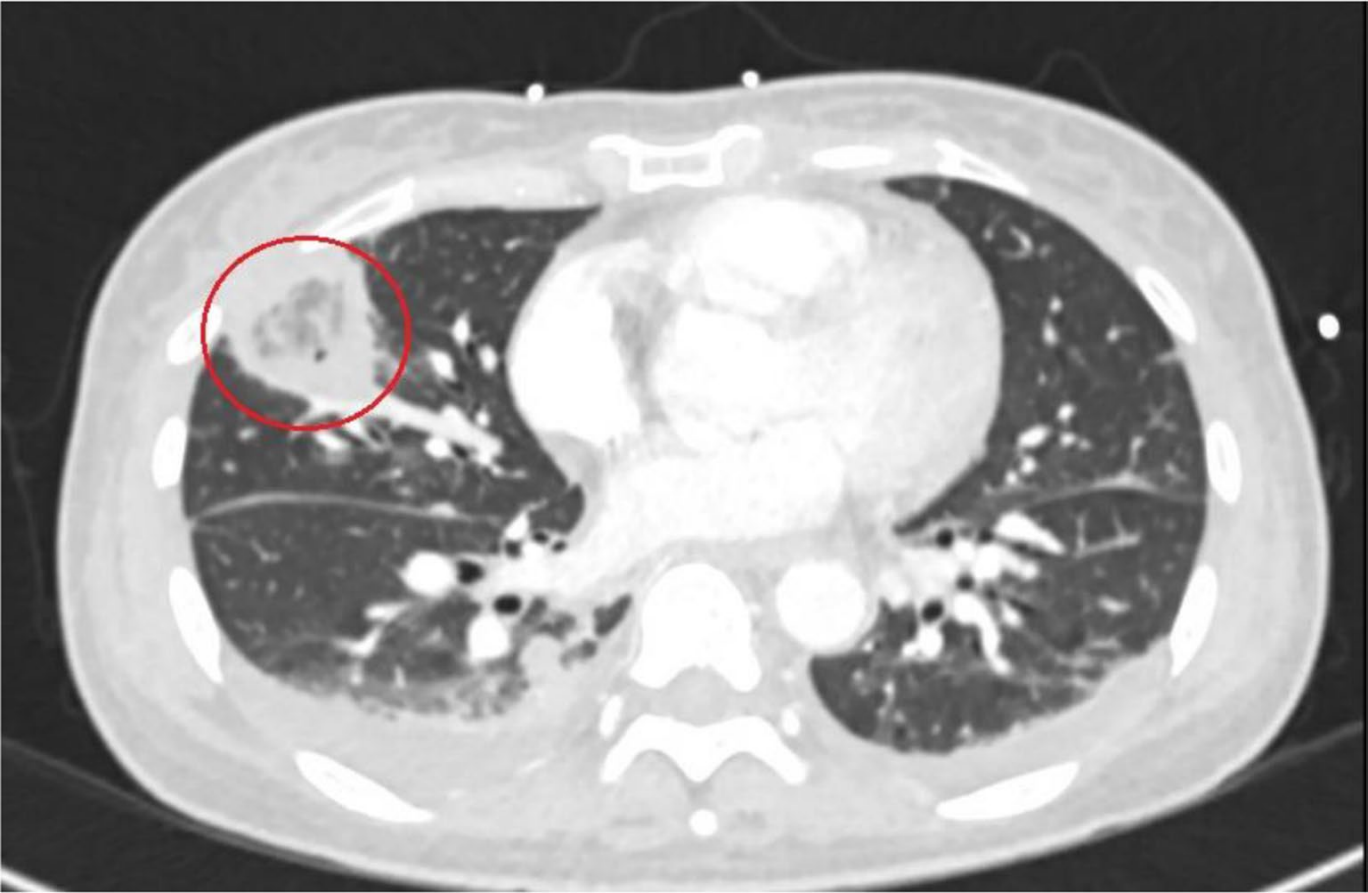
CT scan of the chest at lower thoracic level (day of admission): ground-glass appearance with surrounding consolidation (reversed halo-sign) in the right upper lobe of the lung
